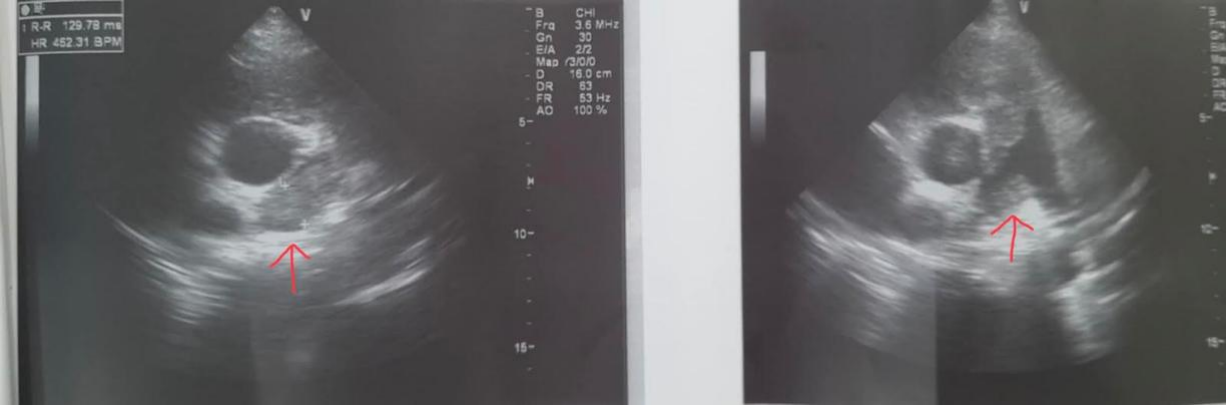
Transthoracic echocardiogram after pericardial and pleural fluid drainage showing complete resolution of the effusion.
